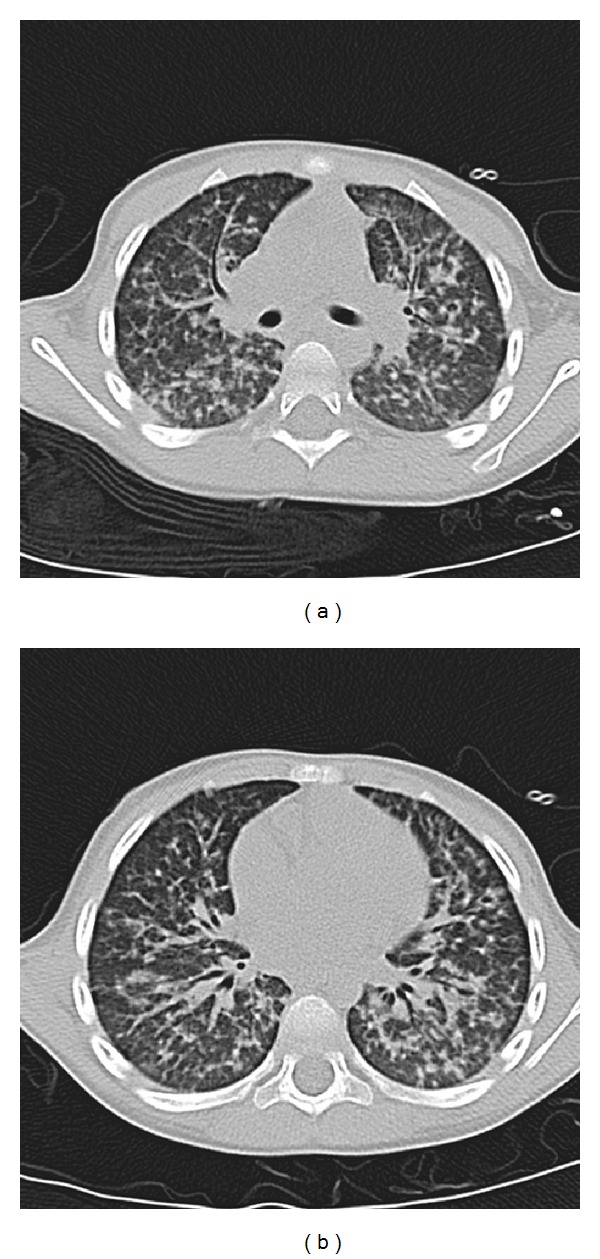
Chest CT prior to admission. Imaging showing diffuse nodular pattern involving different areas of the lungs. There is absence of air trapping or bronchiectatic changes usually seen when there is concurrent involvement of the conductive airways. These images were obtained at the age of 3 years during initial episode of pneumonia.
